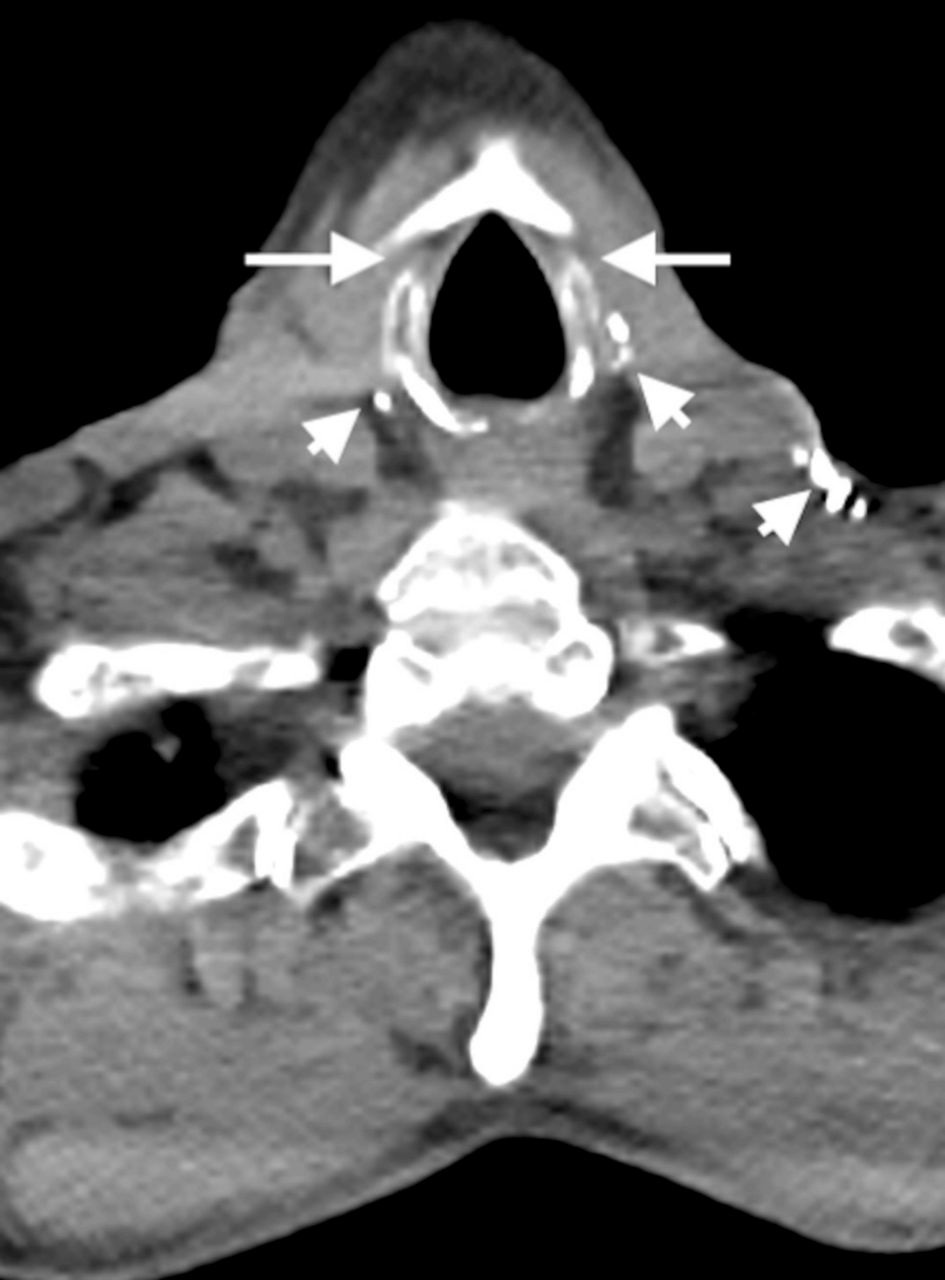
Axial non-contrast CT in soft tissue window from PET/CT through the level of the neck at the inferior thyroid cartilage. Demonstrates a surgically absent thyroid gland in the region of the thyroid bed (long arrows). The thyroid gland would normally appear in this area as a hyperdense butterfly-shaped soft tissue mass. Also noted in the anterior soft tissues of the neck are multiple surgical clips (short arrows) consistent with the patient’s history of total thyroidectomy and cervical lymph node dissection.
